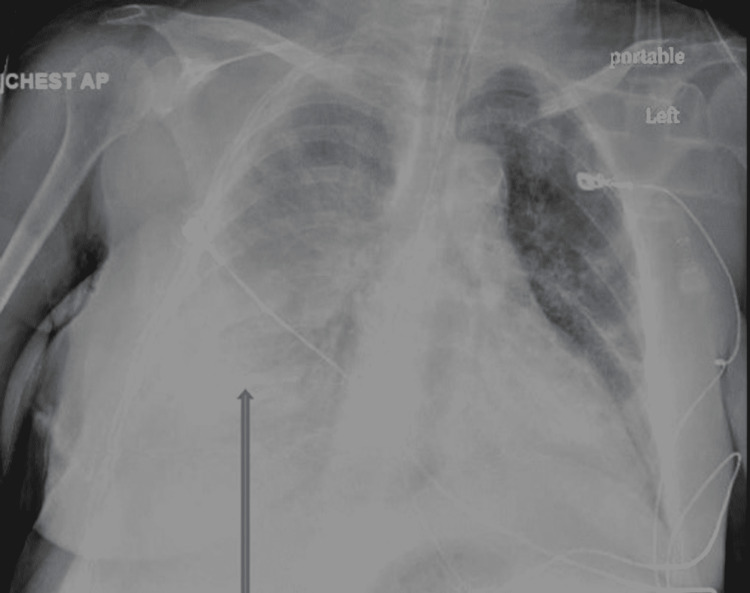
Portable Chest X-ray anteroposterior view showing a white-out right hemithorax suggestive of right hemothorax after DNase/tPA therapy (arrow). DNase: deoxyribonuclease; tPA: tissue plasminogen activator
